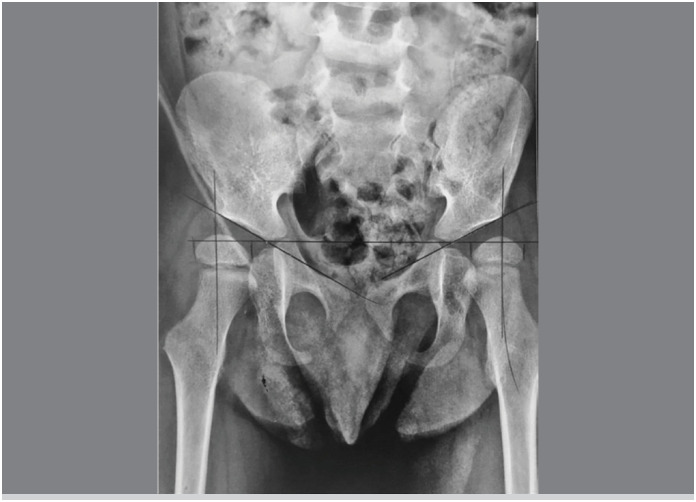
Anteroposterior radiography of the pelvis with migration percentages of the femoral head measurement.
